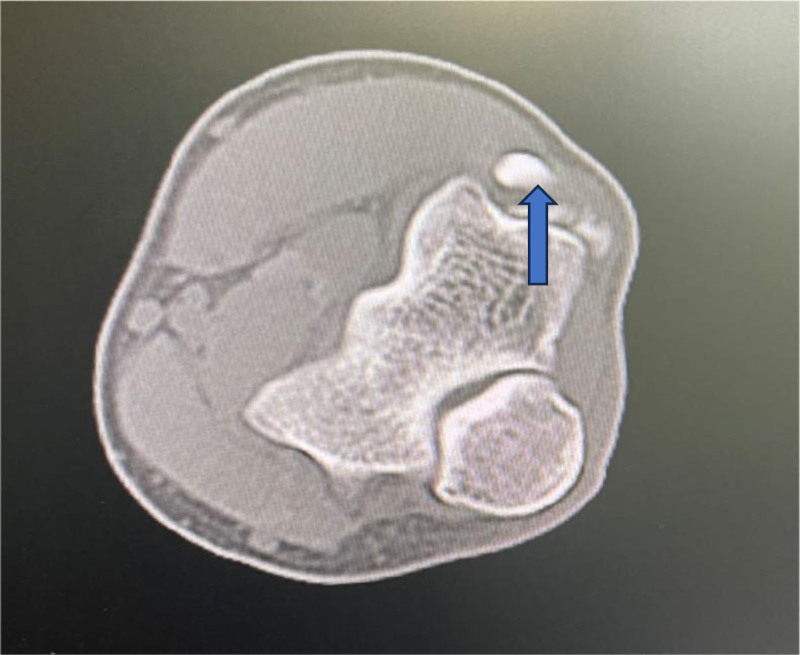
Computed tomography of high-density macular focus and smooth edge of the lateral condyle of the right humerus and dorsal side of the ulnar olecranon.
